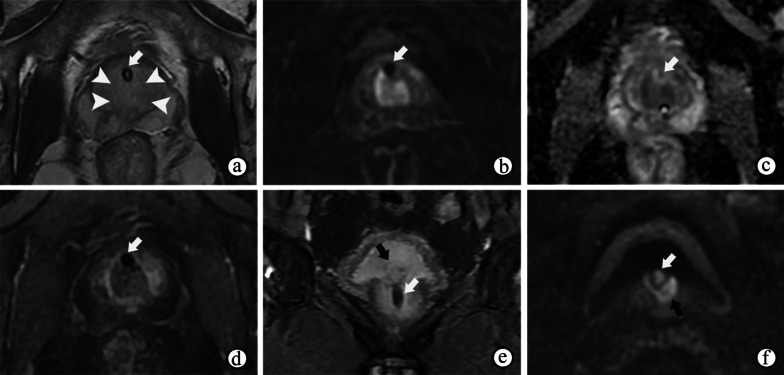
Malignant solitary fibrous tumor originating from the prostatic urethra in a 62-year-old male with a slightly elevated serum PSA level (4.770 ng/mL) and a Foley catheter to relieve urinary retention (white arrow). a T2WI shows a heterogeneously hyperintense nodule (1.8 cm in size) with an obscured hypointense capsule (arrowhead) behind and adjacent to the prostatic urethra in the prostate. b, c DWI at a b-value of 1400 s/mm^2^ and ADC maps show that the nodule exhibits mildly and inhomogeneously restricted diffusion. d DCE imaging shows continuously mild and heterogeneous enhancement of the nodule. e, f Coronal fat-suppressed T2WI and DWI at a b-value of 1400 s/mm^2^ show the nodule protruding into the bladder (black arrow)
